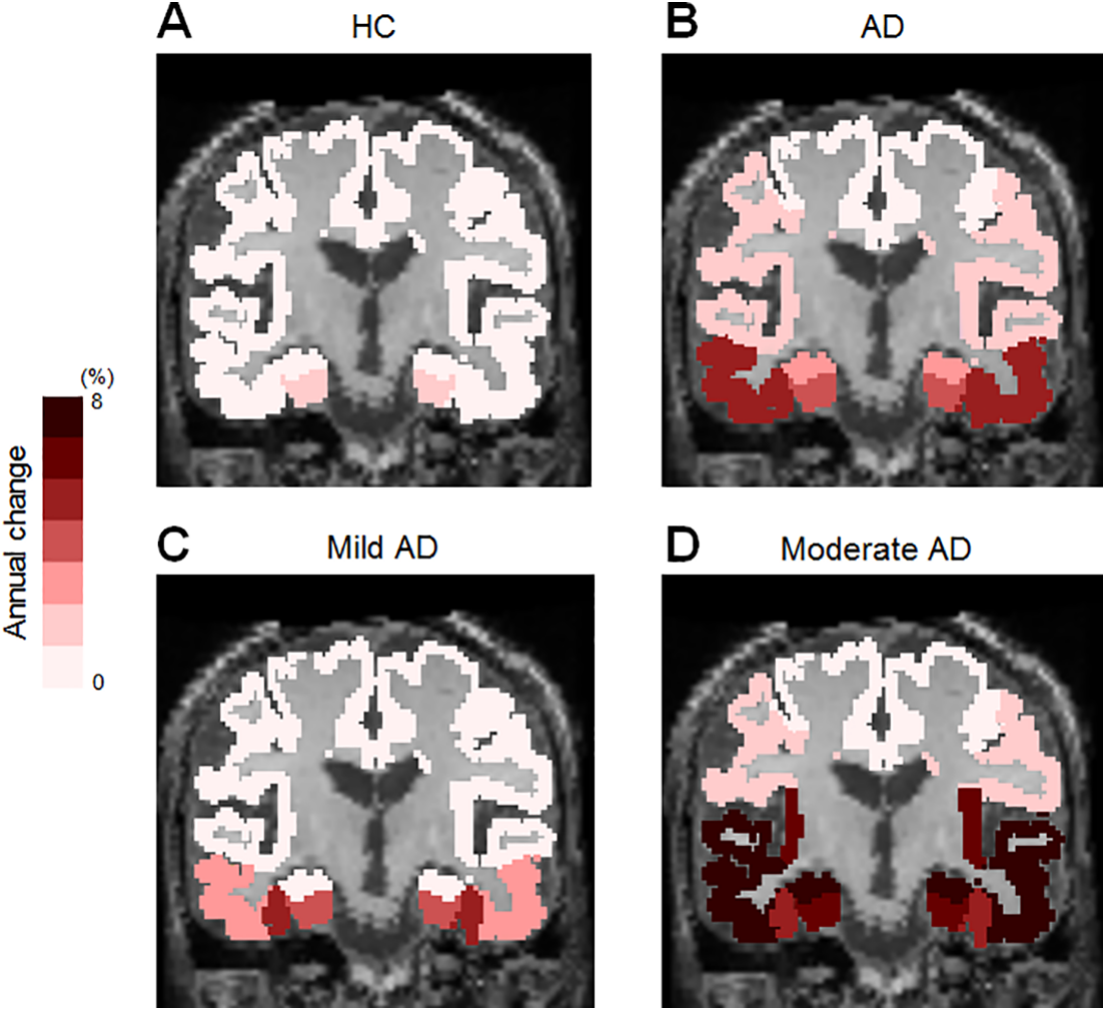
Regional differences in the [^18^F]THK-5117 SUVR annual change in HCs (A) and patients with AD (B). Patients with AD showed greater annual change in the middle and inferior temporal gyri (4.98 ± 3.92%) and in the fusiform gyrus (5.19 ± 2.01%) than did HCs. Compared to the patient with mild AD (57-year-old male, MMSE score of 22 and ADAS-cog score of 12.7 at baseline) (C), the patient with moderate AD (86-year-old female, MMSE score of 18 and ADAS-cog score of 25.3 at baseline) showed greater annual change in the parahippocampal gyrus (7.16%), fusiform gyrus (5.17%), and middle and inferior temporal gyri (8.60%).
